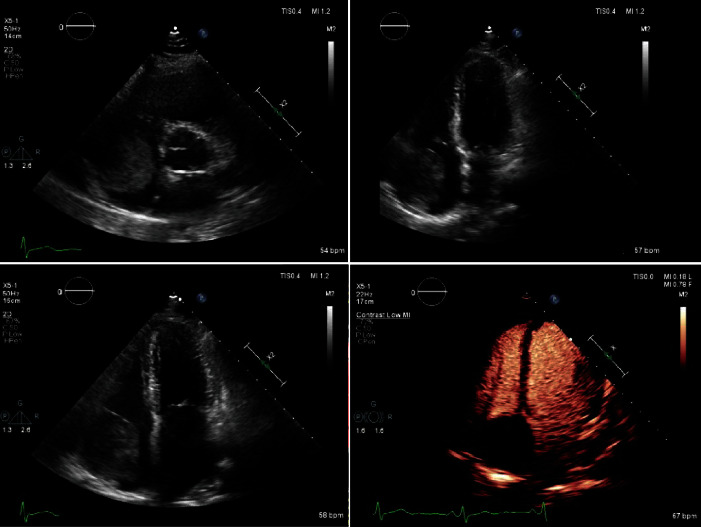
Transthoracic echocardiogram (TEE) view of the mass. Myocardial contrast echo findings suggest minimal to no vascularity within the mass.
